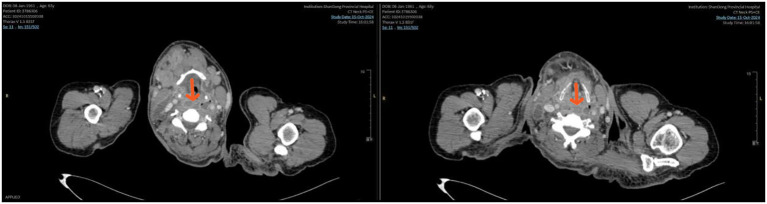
CT scan of patient 2 showing extensive bilateral cervical involvement. Contrast-enhanced CT reveals diffuse soft-tissue swelling of the bilateral cervical and pharyngeal walls, with multiple irregular low-density lesions in the right submandibular region, bilateral cervical spaces, posterior pharyngeal wall, and supraclavicular areas. These findings indicate multiloculated abscesses and widespread deep neck space infection.
